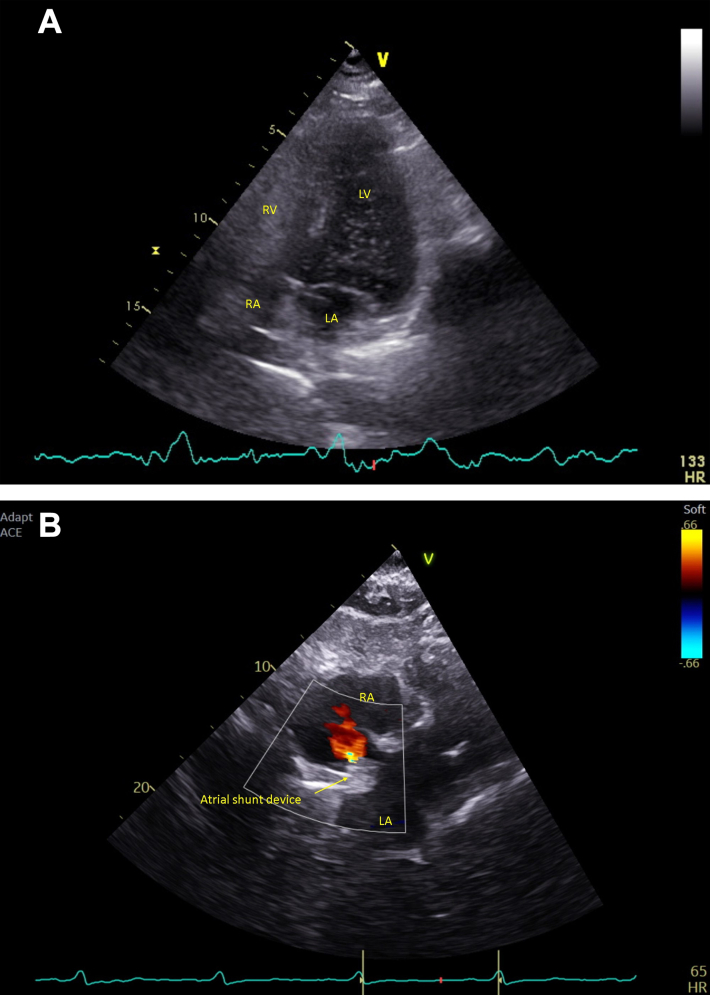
Echocardiographic Findings of Sequential Changes in Shunt Direction (A) Apical 4-chamber view. Right-to-left shunting demonstrated by bubble contrast echocardiography. Bubble contrast echocardiography performed in November 2020 showed right-to-left shunting through interatrial communication. The contrast bubbles appear in the left heart chambers shortly after their injection in the right atrium, confirming the presence of right-to-left shunting. (B) Subcostal view. Left-to-right shunting demonstrated by color flow Doppler echocardiography. Color Doppler echocardiography performed in September 2024 demonstrated left-to-right shunting at the atrial level. The color Doppler signal showed the flow from the left to right atrium, indicating a reversal of the previous right-to-left shunt. Oxygen saturation measurements were normal at this time. LA = left atrium; LV = left ventricle; RA = right atrium; RV = right ventricle.
